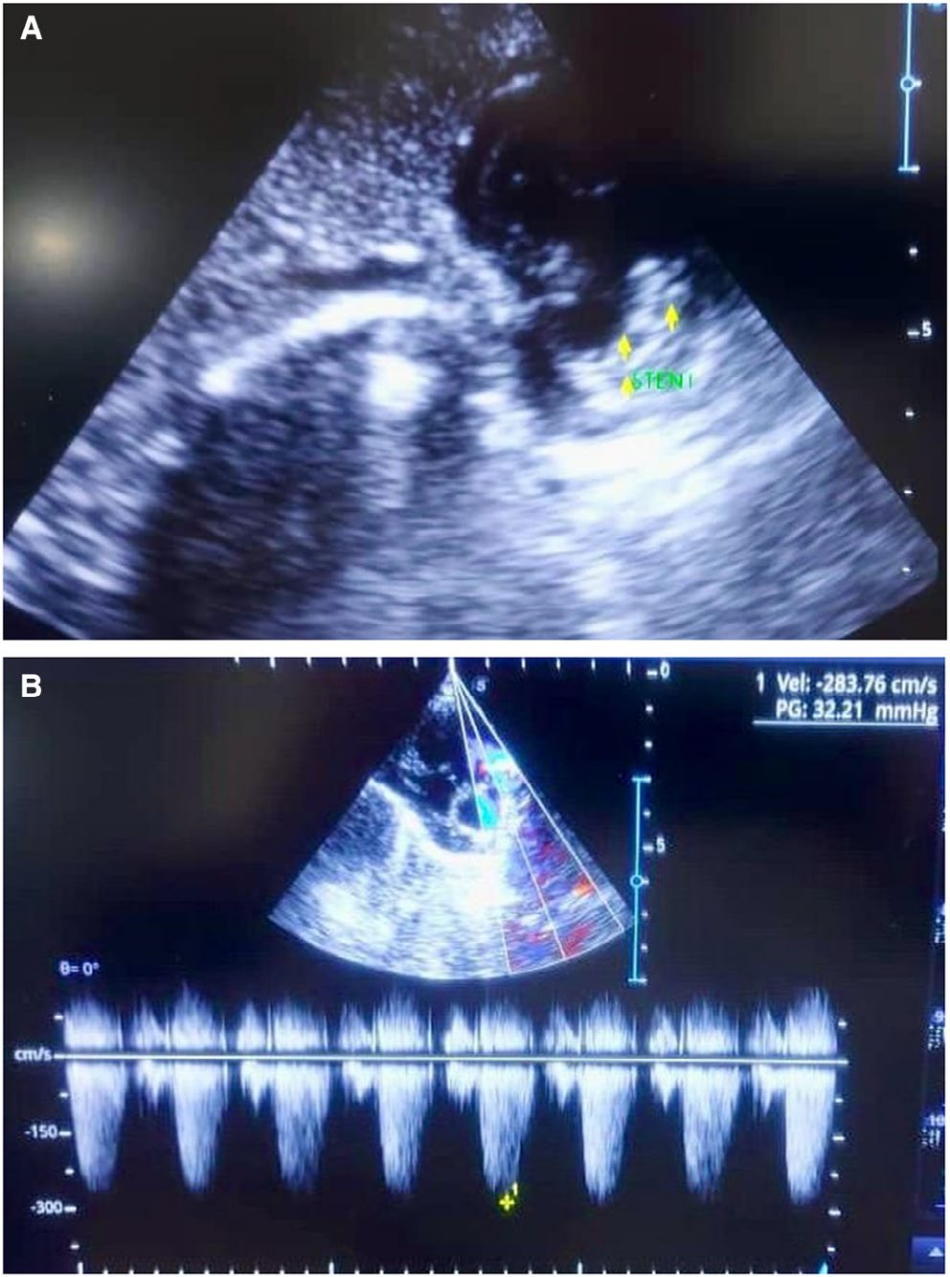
(A and B) Echocardiography 6 months after stent placement surgery.
